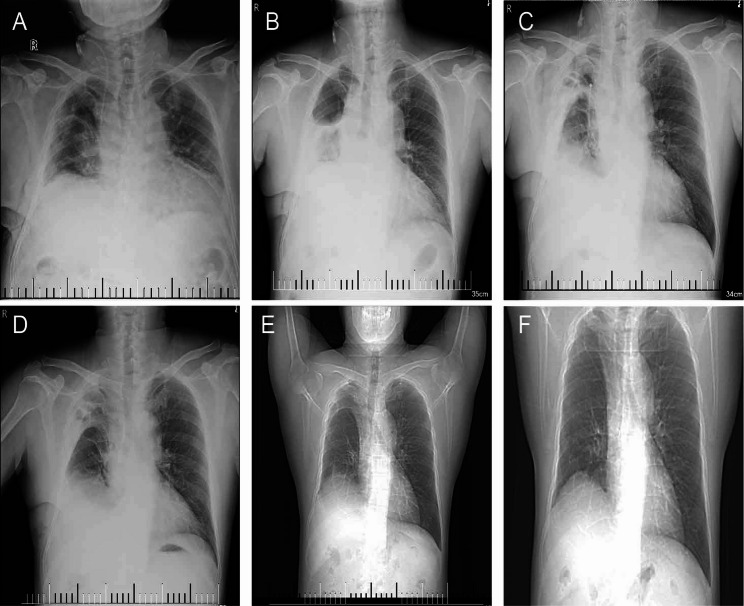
Serial imaging of the clinical course. (A-D) Chest radiographs. (A) Postoperative day 2, showing satisfactory lung re-expansion. (B) Postoperative day 3, showing complete atelectasis 2 h after chest tube removal. (C) After 3 days of conservative treatment, showing radiographic improvement with partial re-expansion. (D) At discharge. (E-F) CT topograms (scout views). (E) Three months postoperatively, showing complete re-expansion of the residual right lung. (F) Two years postoperatively, demonstrating sustained lung expansion without recurrence
